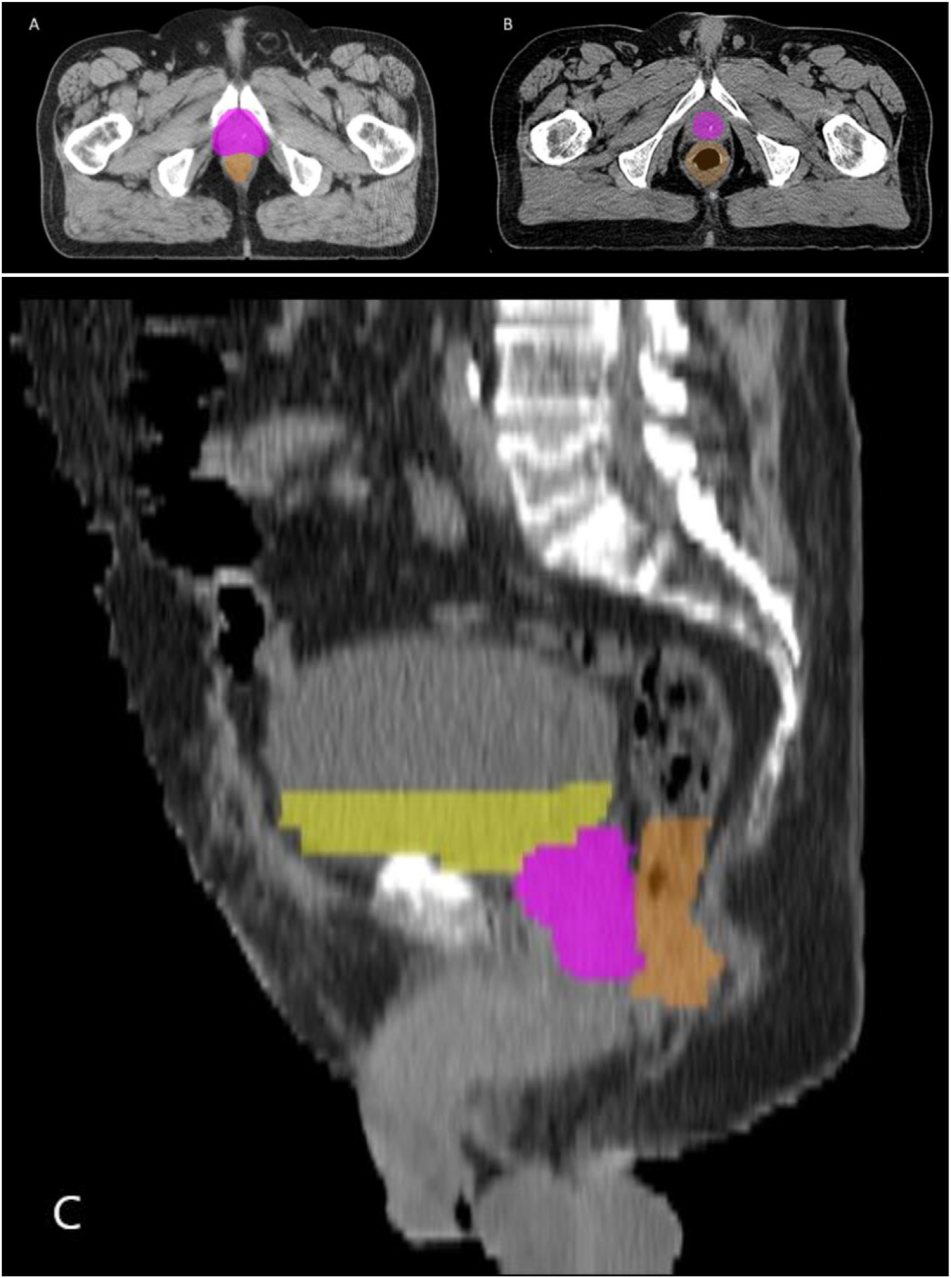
Pretreatment computed tomography scans of 3 representative patients with prostate cancer who did not meet the criteria of acceptance and were excluded from the training process. The colored delineations in yellow, pink, and orange correspond to manual contouring of the urinary bladder, prostate, and rectum, respectively. (A) and (B) Transverse plane of 2 patients from the initial cohort. The prostate delineation of patient A overlapped with nearby structures, especially anteriorly. The manual delineation of the prostate in patient B did not cover the whole organ. (C) Sagittal plane of a patient from the initial cohort. This case was excluded because large parts of the urinary bladder and rectum contouring were missing.
